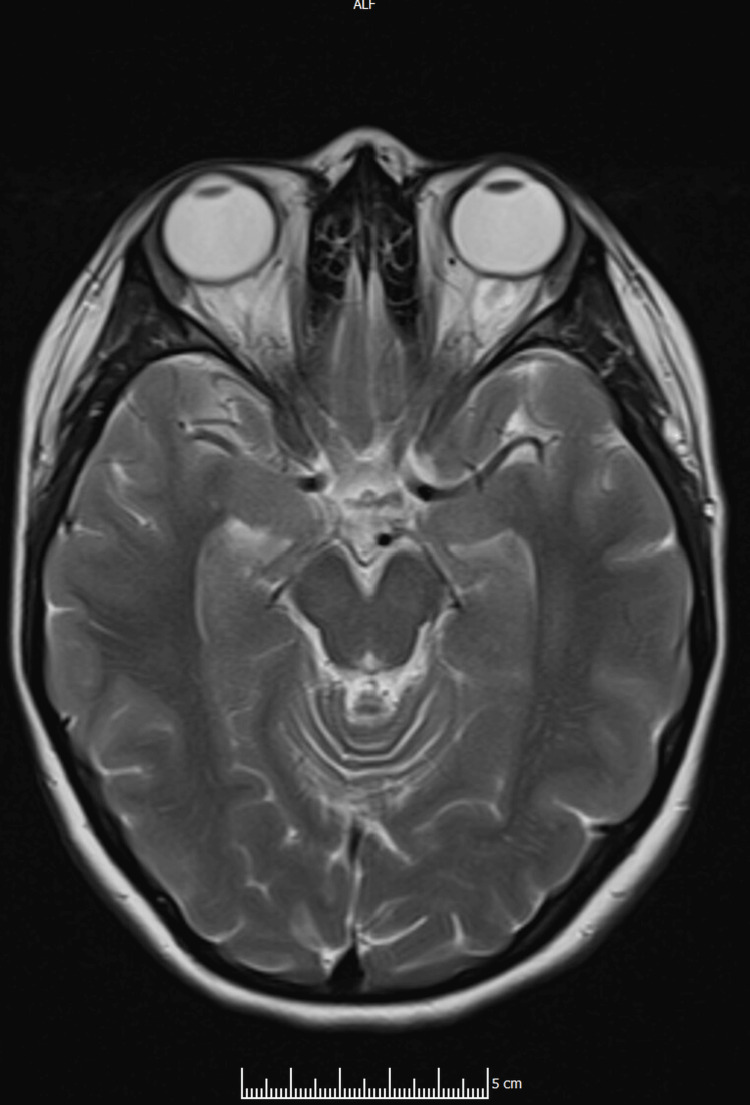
Axial orbital MRI (T2): posterior scleral flattening and perioptic subarachnoid space distension MRI: magnetic resonance imaging
